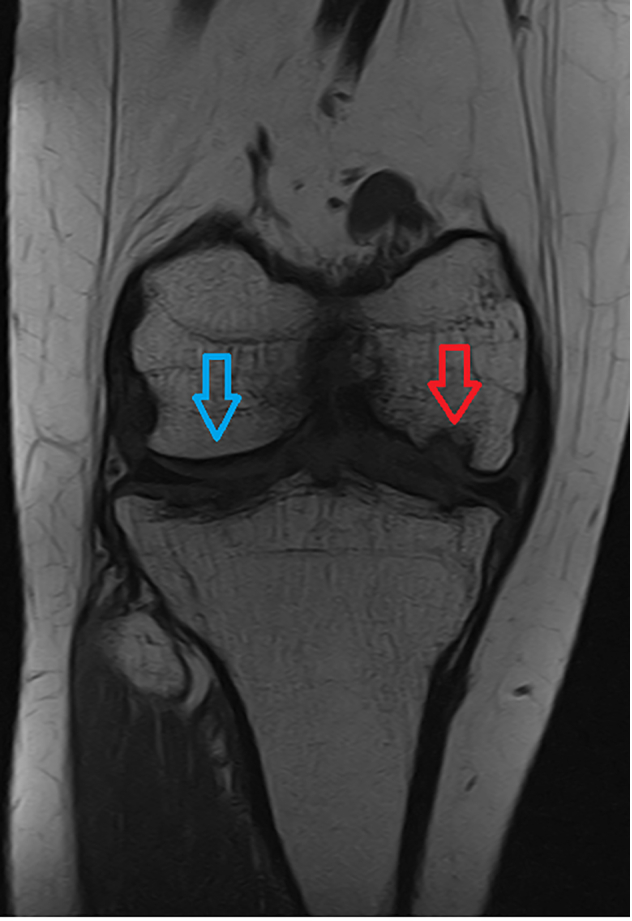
T1 weighted coronal MRI image showing extensive defect of medial femoral condyle in comparison with relatively normal lateral femoral condyle. Red arrow indicates medial lesion. Blue arrow indicates noral lateral femoral condyle.
